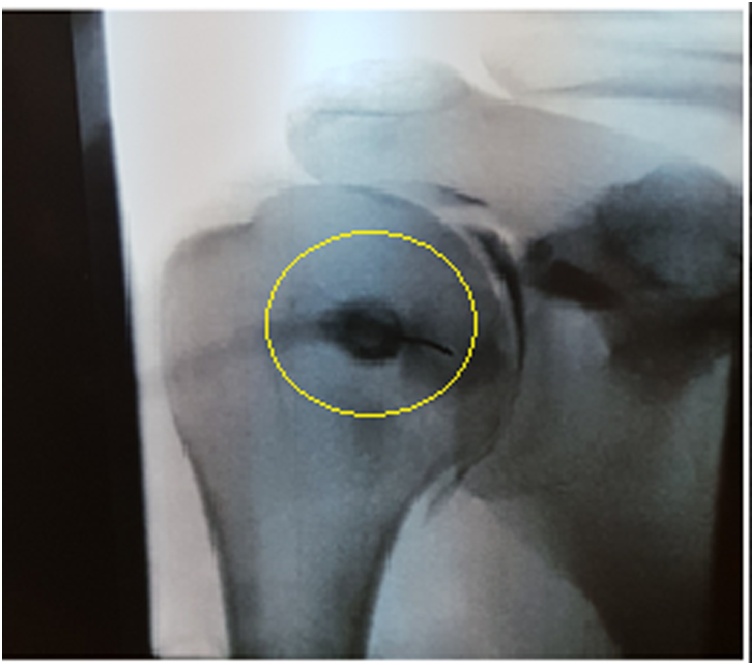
X-ray films under fluoroscopy with intraarticular contrast showing cyst and fluid extravasation.
